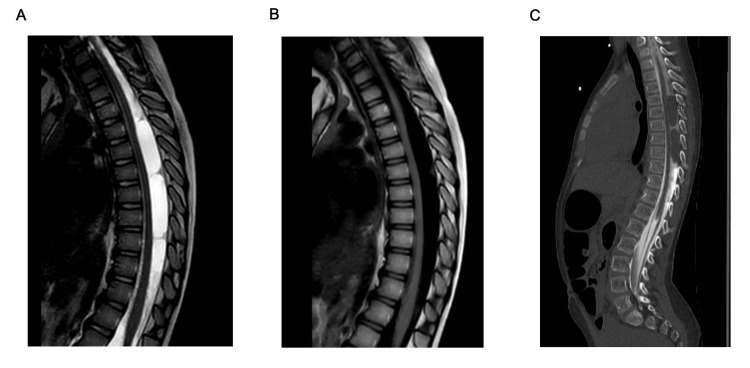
Sagittal MRI thoracic spine, sagittal T2 (A) and T2 flair (B), demonstrate imaging characteristics consistent with cerebrospinal fluid. CT myelogram (C) images showing interruption of normal contrast flow at the mid-thoracic level due to mass effect from the arachnoid cysts.
